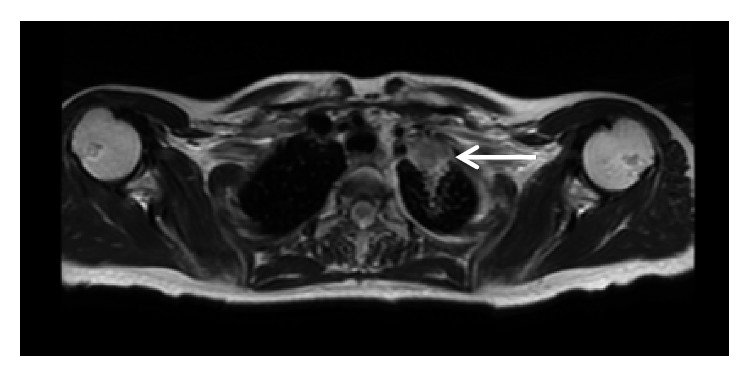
MRI of the chest demonstrating a new left apical pleural mass (white arrow).
